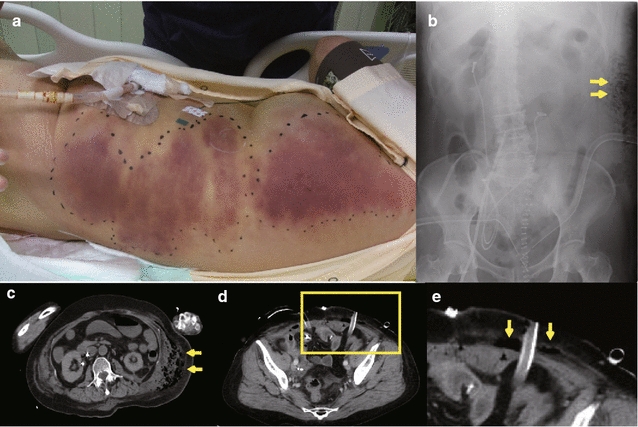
Images of the patient. a A diffuse skin rash developed over the* left side* of her trunk 2 days after the surgery. b Abdominal X-ray taken immediately after ICU admission showed soft tissue swelling with the density of air on the left abdominal wall (*arrows*). c Computed tomography of the abdomen taken 2 days after the surgery showed subcutaneous emphysema over the* left side* of the trunk (*arrows*). d Enhanced computed tomography of the abdomen taken 1 day after the surgery. The *rectangle* indicates the area shown in e. e The *arrows* indicate air-densities between the subcutaneous fat tissue and muscle layer in the vicinity of the left drainage tube
